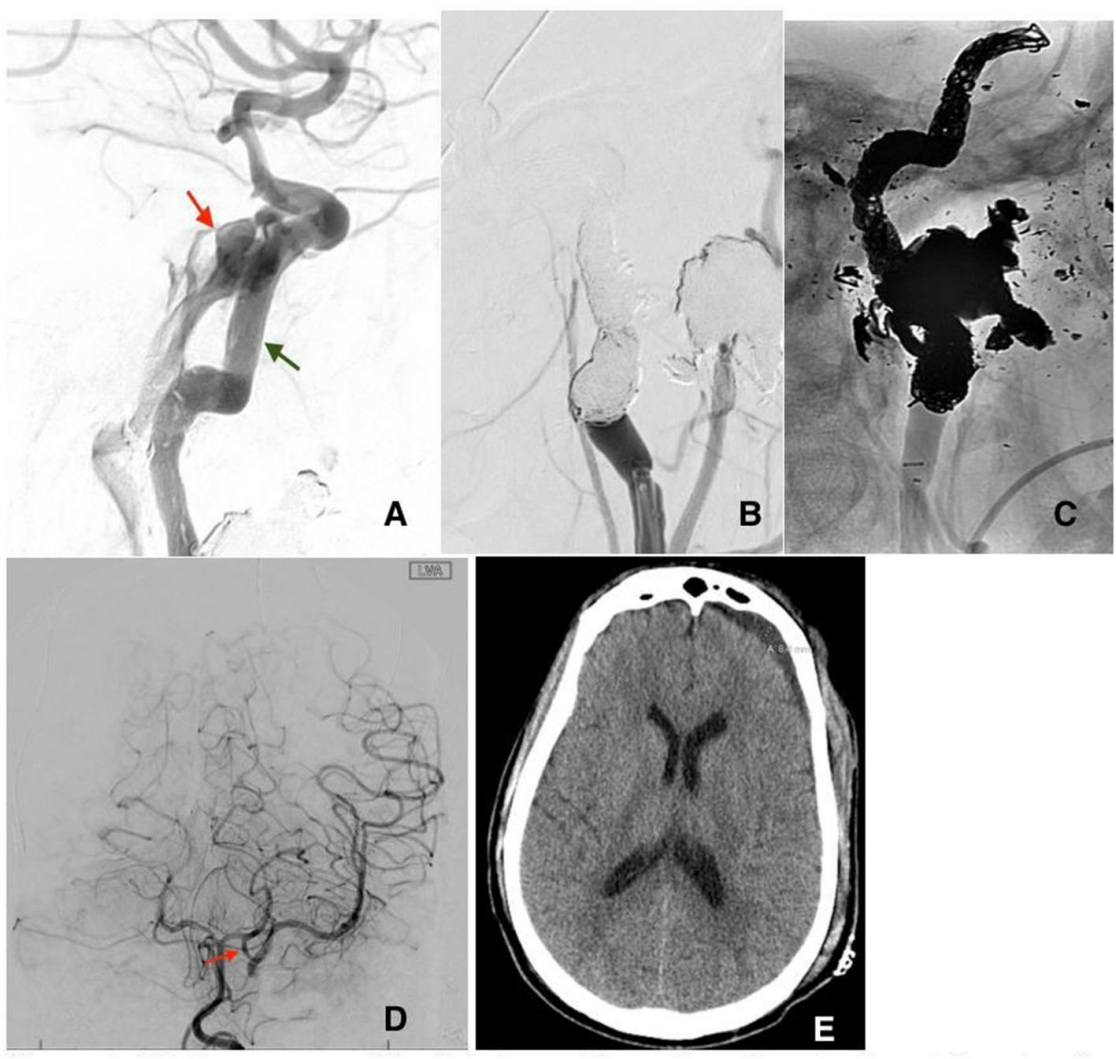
(A) Recurrence of the fistula post flow diversion stenting at the site of the ruptured pseudoaneurysm (red arrow). Notice the misplacement of the previously deployed stents (green arrow) secondary to the high flow of the CCF. Subtracted (B) and unsubtracted (C) views of the left ICA post sacrifice by means of coiling without any residual flow in the CCF. (D) Cerebral angiogram of the left vertebral artery post left ICA sacrifice AP view, showing adequate flow into the left middle cerebral artery through a large left posterior communicating artery (red arrow). (E) Head CT before discharge showing residual subdural hematoma with no signs of ischemic changes in the left hemisphere.
